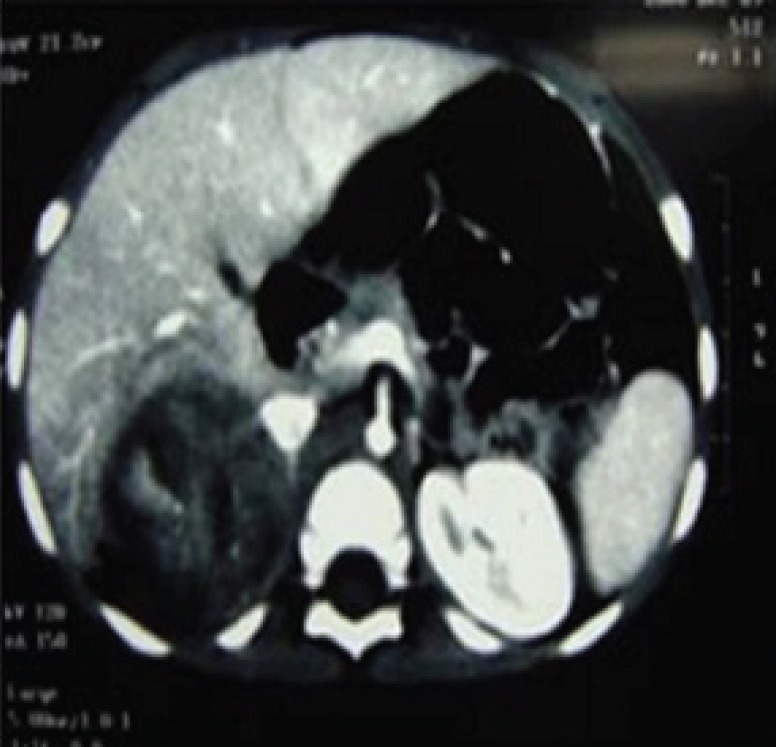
An abdominopelvic CT scan image shows a large oval shaped heterogeneous mass arising from the right adrenal gland with heterogeneous enhancement and pressure effect on right lobe of the liver.
